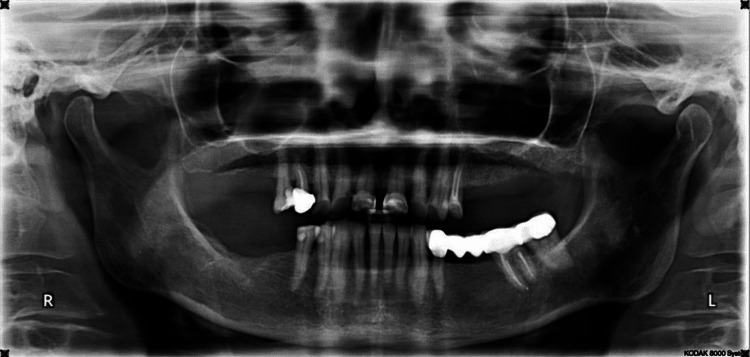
A 64-year-old female patient; orthopantomography
